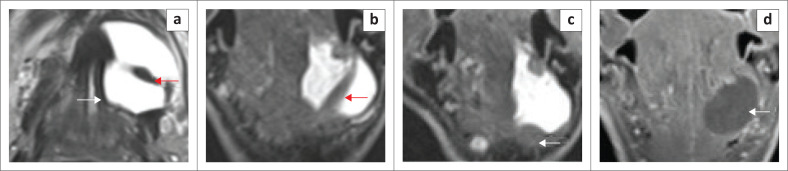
A ranula in an 85-year-old patient who presented with a painless swelling in the left floor of the mouth. MRI neck T2-weighted (T2W) axial (a) T2-weighted short-tau inversion recovery (T2W-STIR) posterior coronal image (b) reveal the left floor of the mouth simple cystic mass dissecting through the left mylohyoid muscle (red arrow) and indenting the left geniohyoid muscle (a, white arrow). The anterior coronal T2W short-tau inversion recovery (STIR) image (c) shows the cyst limited by the anterior belly of digastric muscle inferiorly (white arrow). No enhancement of the cyst wall or intracystic enhancing component on the contrast-enhanced coronal T1 fat-suppressed image (d).
